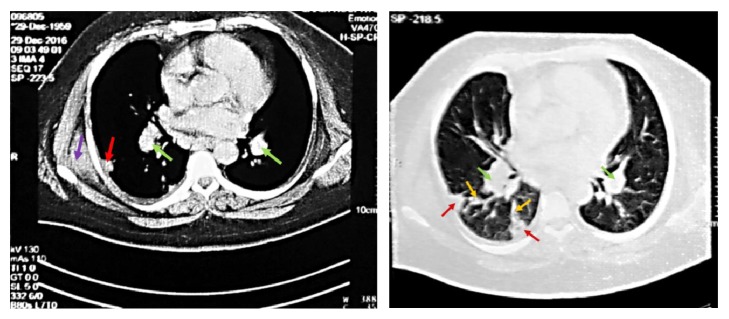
CT of Chest. Lung lesions. Hilar adenopathies (green arrows), probable septic embolization (red arrows), feeding vessels (yellow arrow), and serratus anterior muscle (purple arrow).
